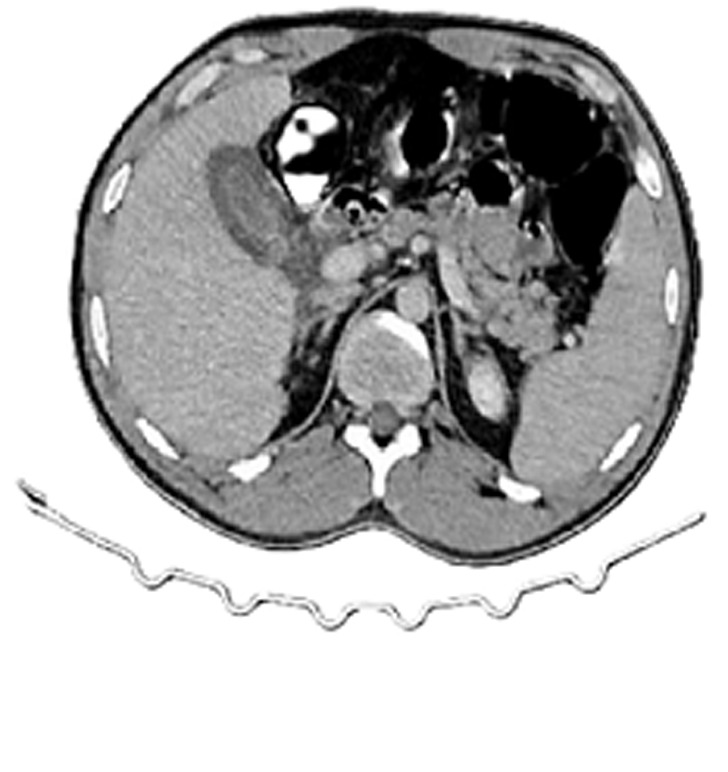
axial computed tomography image showing a gallbladder with diffuse wall thickening, suggestive of ischemia
